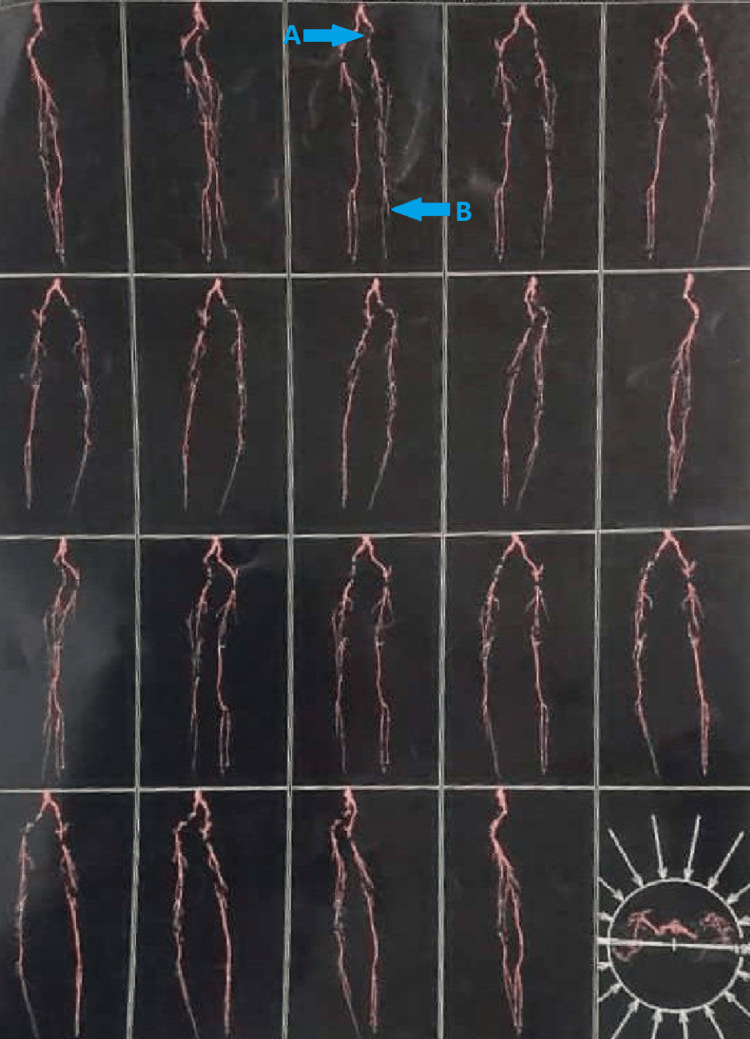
Arterial study of left lower extremity, done through multislice 3D CT angiography of both legs which shows impression (A) narrowing of artery and thrombus seen in left iliac artery, common femoral, superficial femoral artery, popliteal artery, and anterior and posterior tibial artery. For which patient was operated for toe amputation (B) absent flow of blood in left dorsalis pedis artery, first metatarsal artery and dorsal metatarsal arteries of left foot shown by the blue arrow. 3D- 3-dimensional. CT- computerized tomography.
